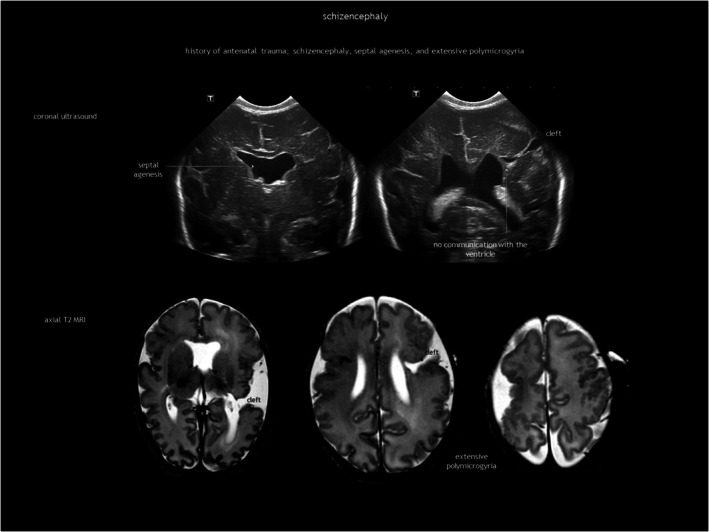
Type 3 schizencephaly. Abbreviation: MRI, magnetic resonance imaging.
